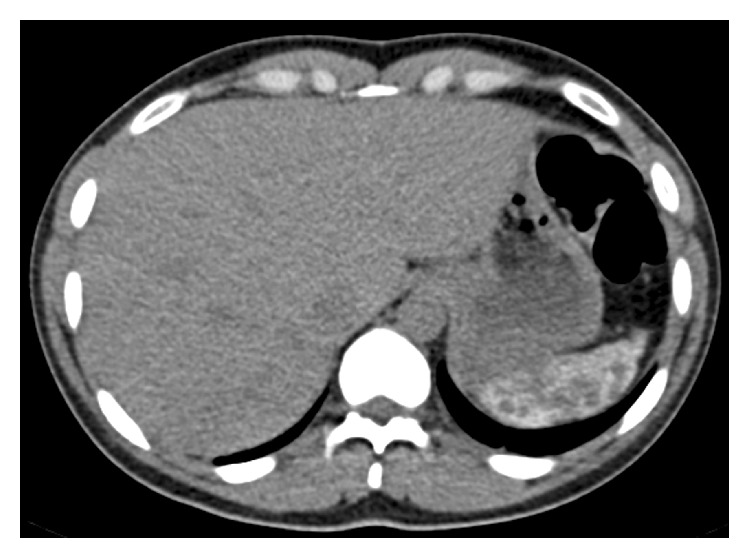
Computed tomography scan section, depicting diffuse calcifications of the spleen.
